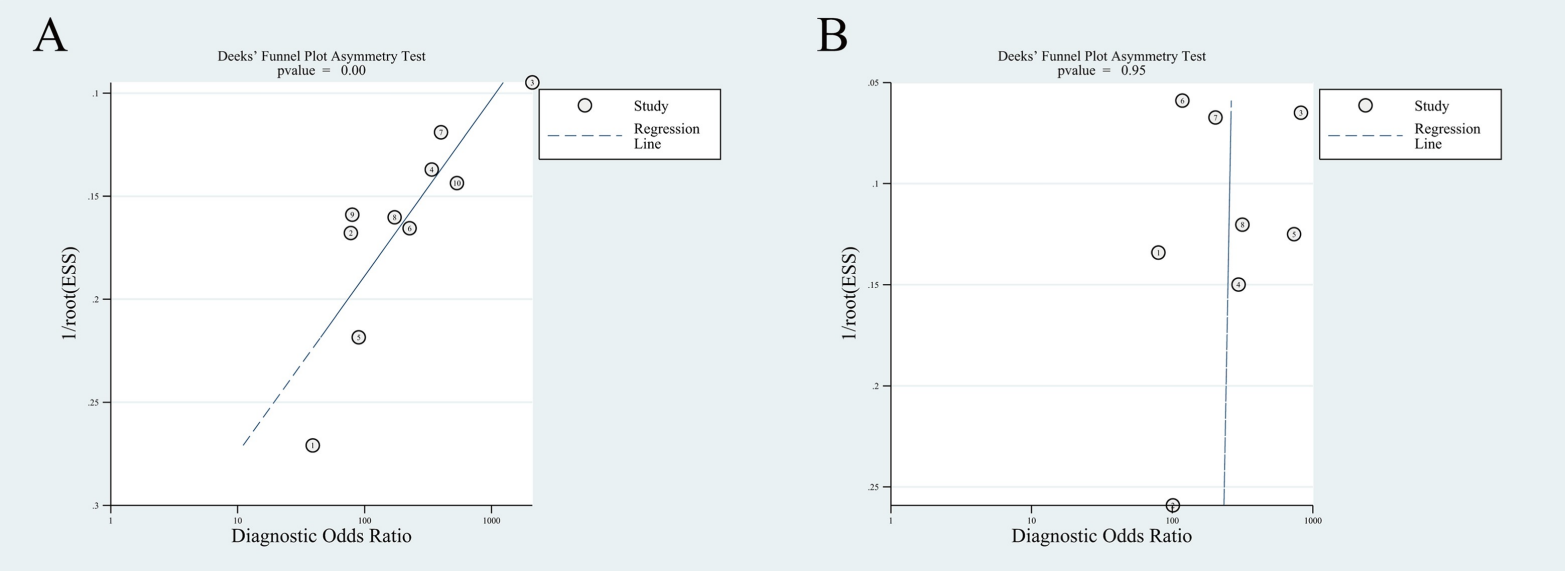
Deeks’ funnel plot test of choline PET/CT on a per-patient basis (A) and on a per-lesion basis (B).
